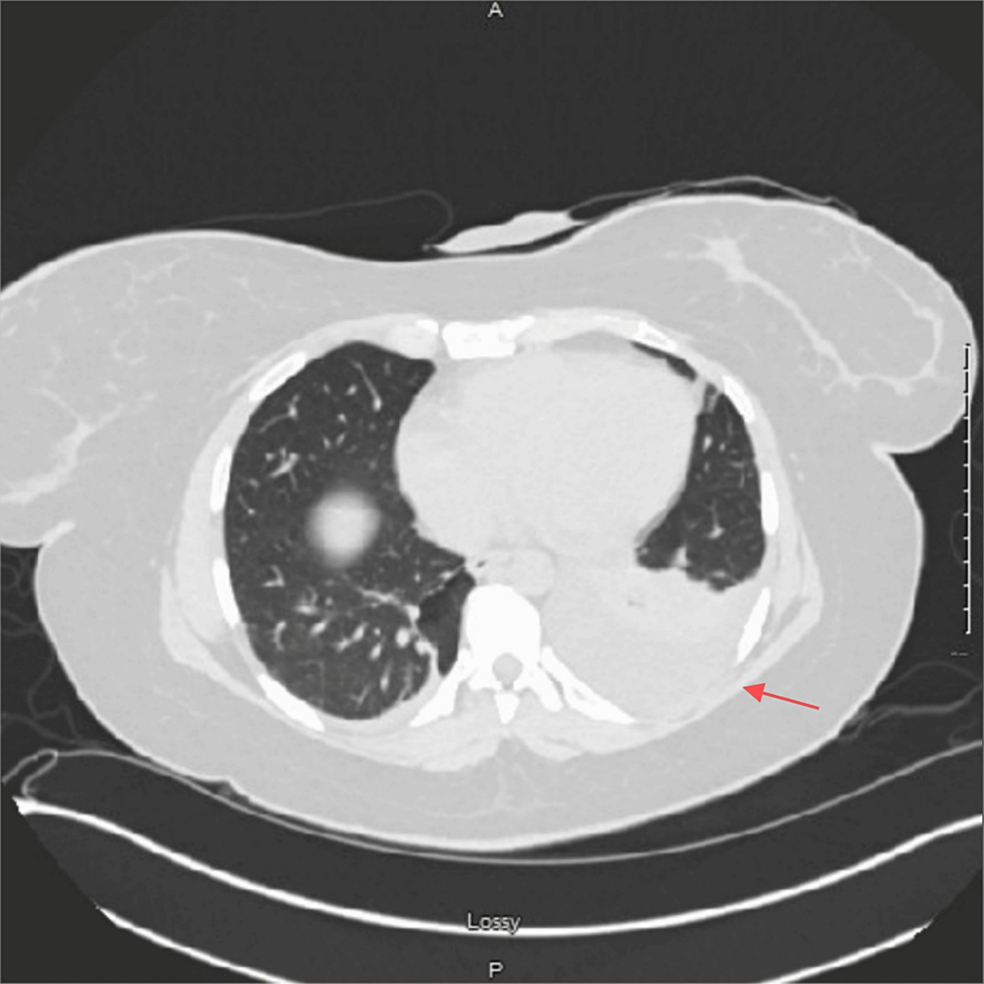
CT abdomen/pelvis without contrast The right arrow indicates left-sided pleural effusion.
